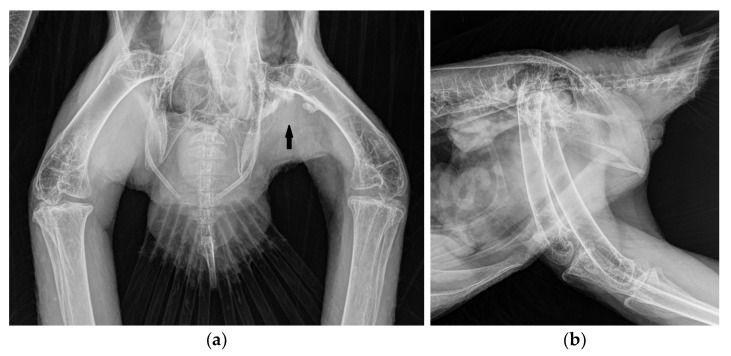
Ventro-dorsal (a) and latero-lateral (b) radiographs of the bald eagle of case 1 with heterotopic bone formations with a rough surface between the femur and pelvis, along the course of the adductors (arrow).
